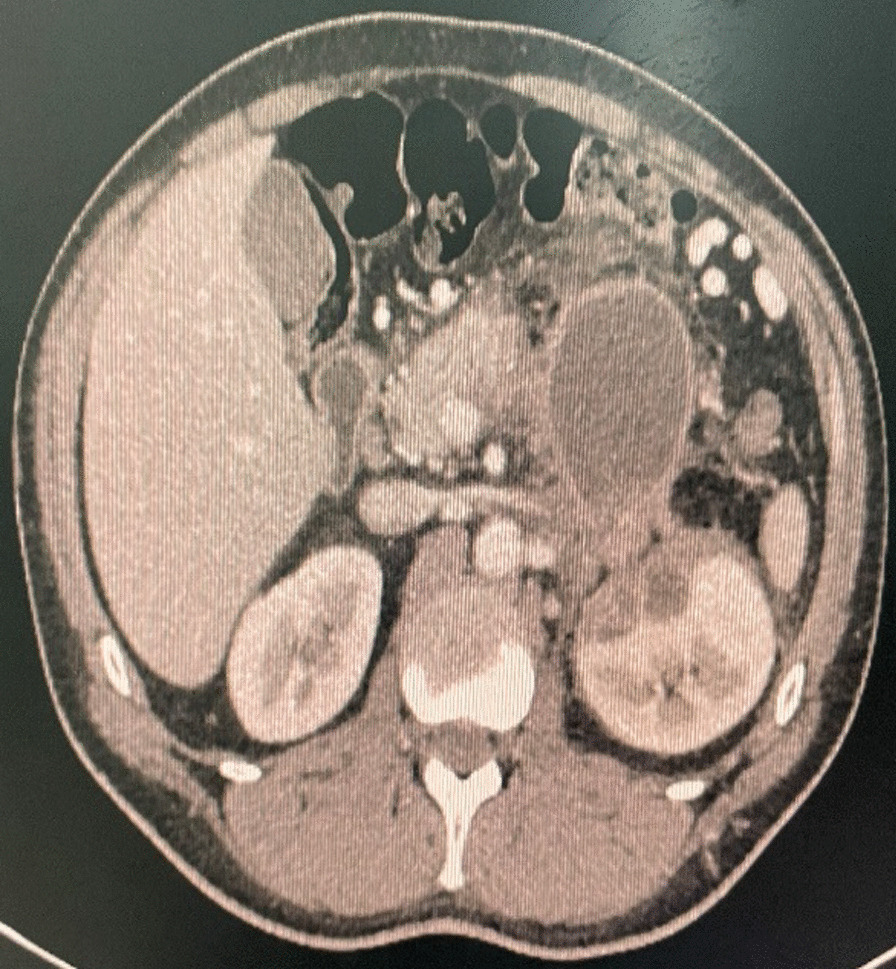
Axial sections of CT scan showing a 20 × 18 mm left renal pseudocyst
